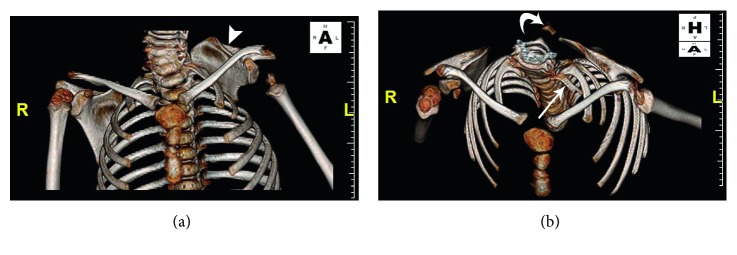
CT, 3D reconstructed images demonstrate Sprengel deformity in a patient with Klippel–Feil syndrome with abnormal elevation of the left scapula (a, arrowhead) and associated omovertebral bone (b, curved arrow). Incidental left cervical rib was also identified (b, arrow). (*Courtesy of Miguel Flores*, *MD*, *Orlando*, *FL.*)
